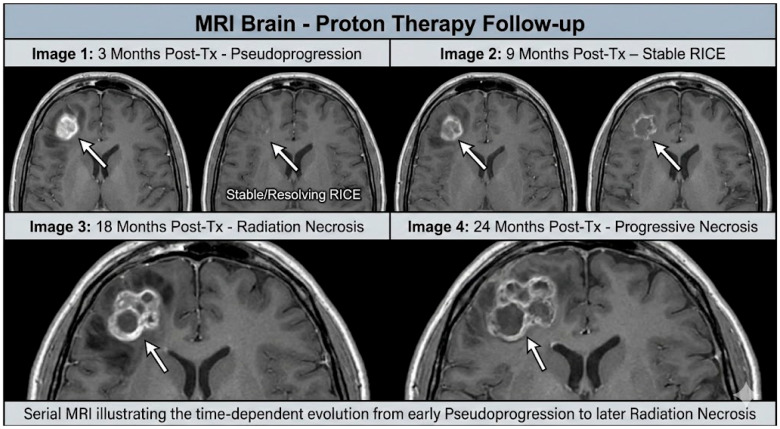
Longitudinal MRI evaluation of RICE, pseudoprogression, and radiation necrosis following proton therapy. RICE: Radiation-induced contrast enhancement.
